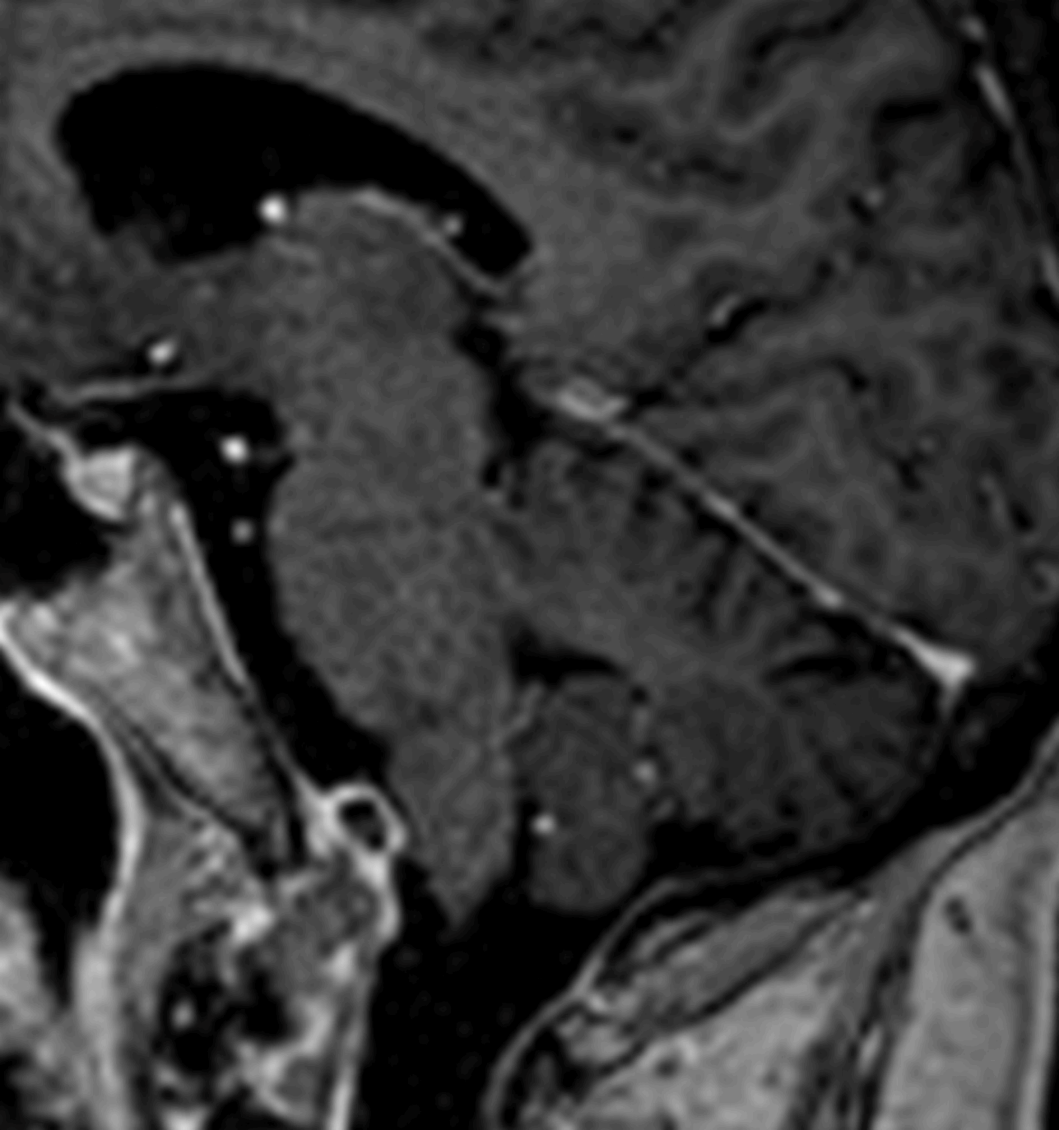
Sagittal, contrast-enhanced T1-weighted MR-image showing a mass (7 × 8 × 15 mm, height x depth x width) with peripheral contrast-uptake that was later identified as a low-grade chondrosarcoma. This mass was attached to the clivus and was located in close proximity to the medulla oblongata. Note that this MR-image was taken on day 2 and remained stationary over the course of the next 2 months.
